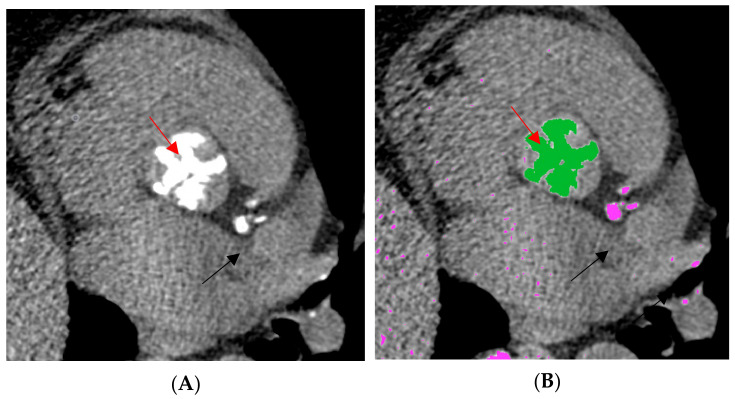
Sample images of native computed tomography showing aortic calcification with the semi-automatic detection of aortic valve calcification with an attenuation threshold of ≥130 Hounsfield units. (A): A two-dimensional representation of the aortic valve in non-contrast computed tomography. (B): A semi-automatic quantification for the Agatston calculation; green = the semi-automatic selection of the aortic valve; pink = detections of calcification at a predetermined threshold of ≥130 Hounsfield units; red arrow = aortic valve; black arrow = calcified structures such as coronary arteries.
